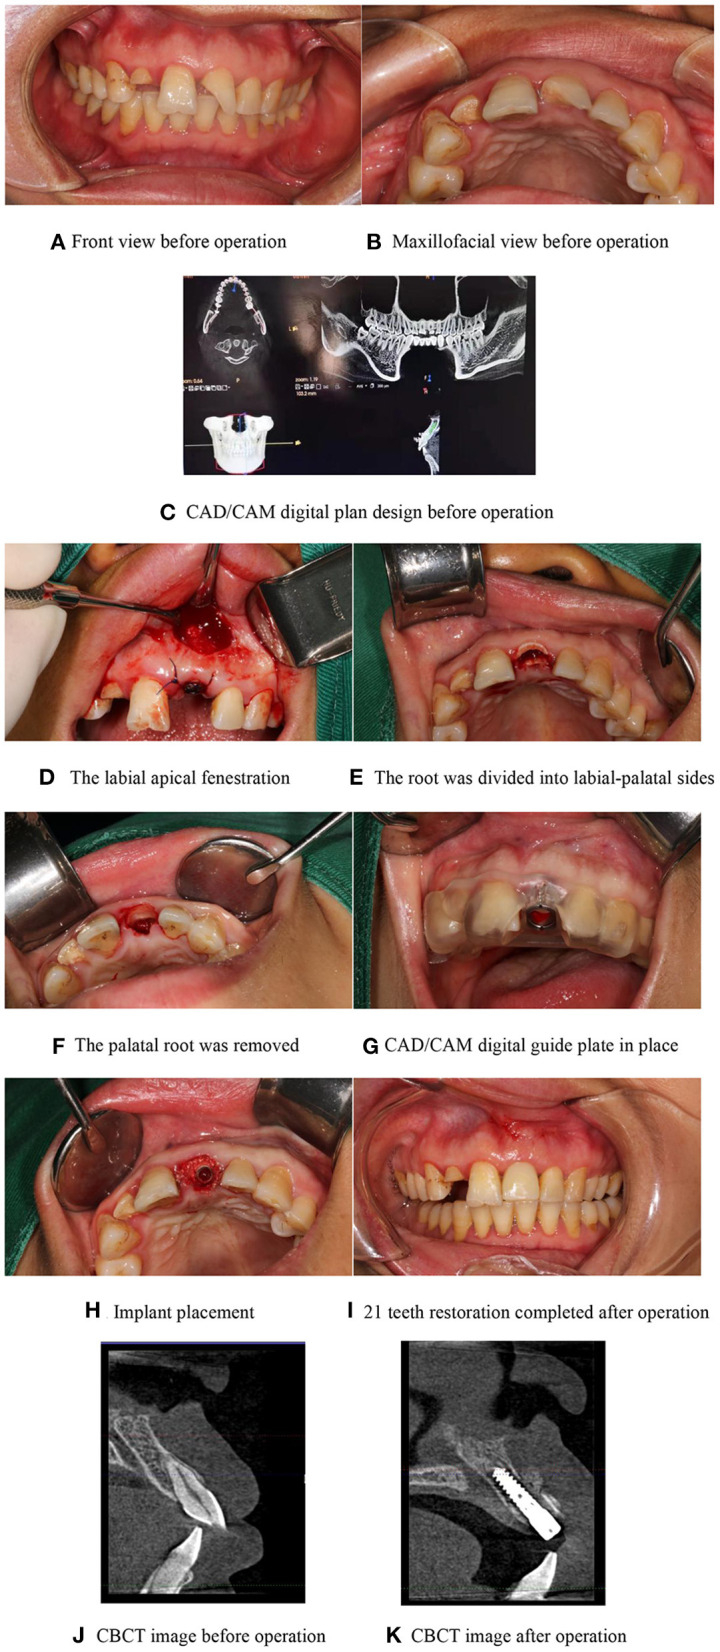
Typical cases. (A) Front view before operation, (B) Maxillofacial view before operation, (C) CAD/CAM digital plan design before operation, (D) The labial apical fenestration, (E) The root was divided into labial-palatal sides, (F) The palatal root was removed, (G) CAD/CAM digital guide plate in place, (H) Implant placement, (I) 21 teeth restoration completed after operation, (J) CBCT image before operation, and (K) CBCT image after operation.
